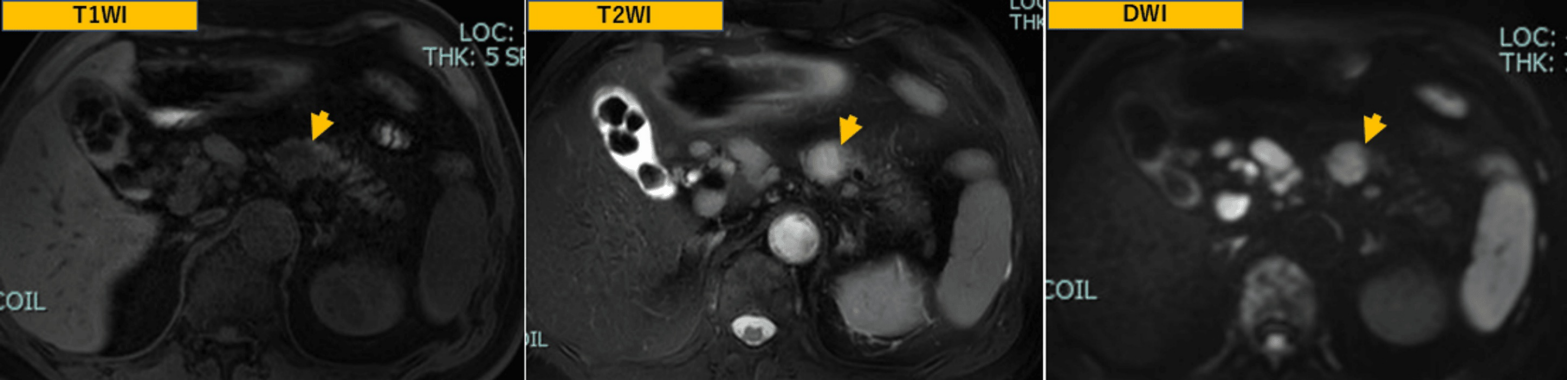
MRI images MRI demonstrated low intensity on T1-weighted images, mildly high intensity on T2-weighted images, and slight restriction on diffusion-weighted imaging of the mass (arrow head).
